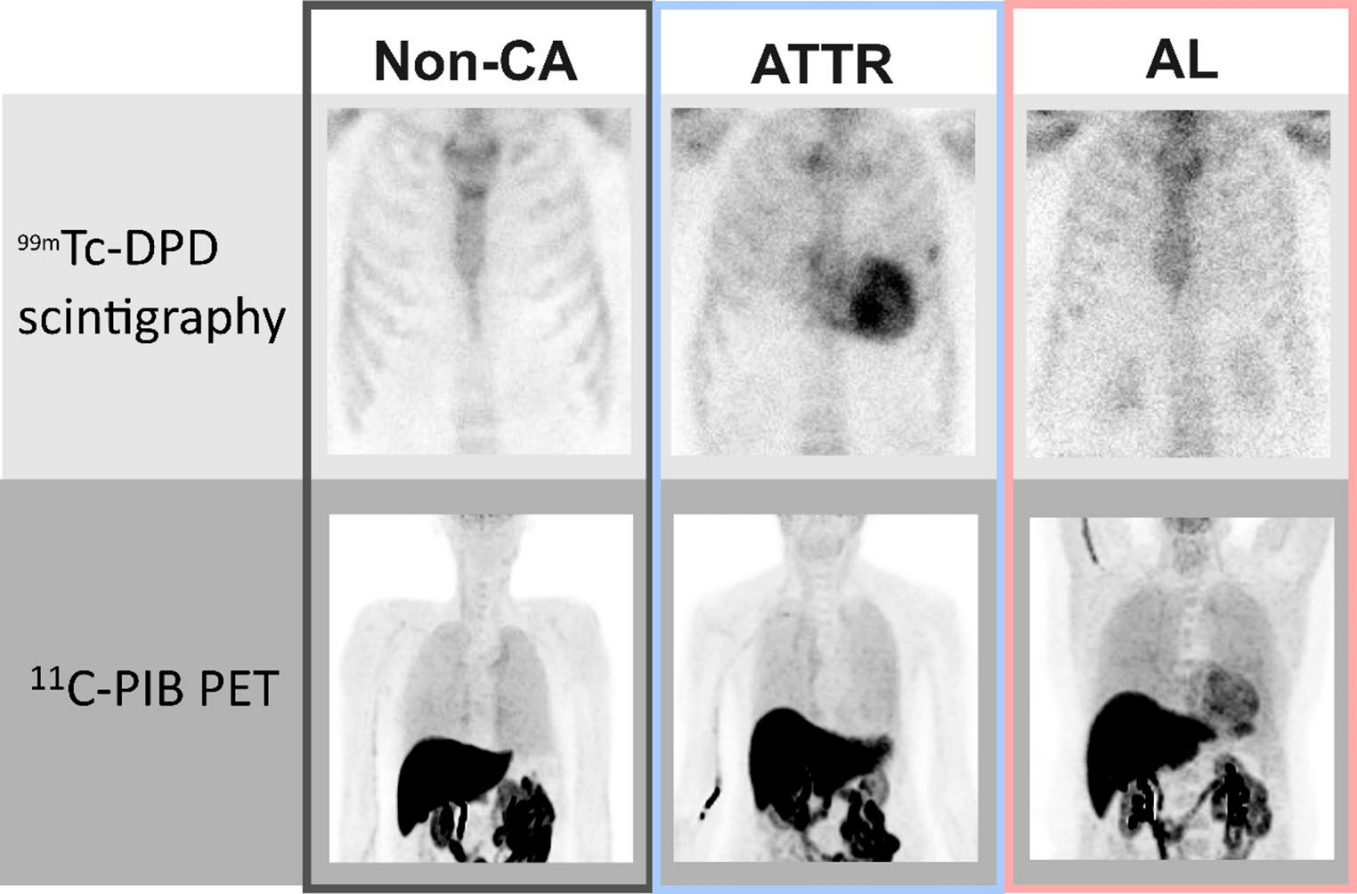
Example images for patients with ATTR, AL, and Non-cardiac amyloidosis. The top row represents scintigraphy images acquired with ^99m^Tc-DPD, the lower row represents PET images acquired with ^11^C-PiB. The left column shows a patient without cardiac amyloidosis (Non-CA), the middle column shows a patient with ATTR and the right column shows a patient with AL
